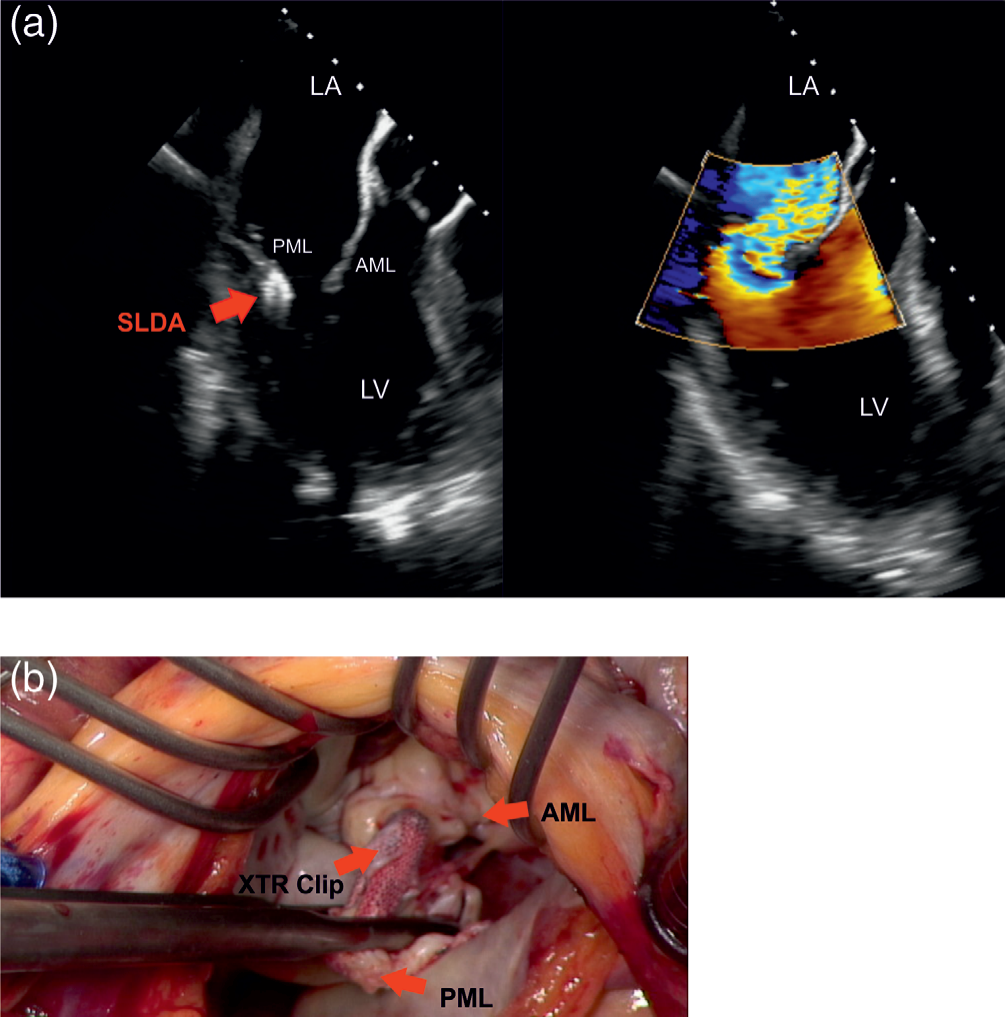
Single leaflet device attachment (SLDA). (A) Shows transesophageal echocardiographic imaging of a patient with SLDA. The MitraClip XTR device (red arrow) is exclusively attached to the posterior mitral leaflet (PML) causing severe MR. (B) Demonstrates an intraoperative image taken during surgery in the same patient. From an atrial perspective one can identify the mitral valve and the MitraClip XTR device held by the surgeon
